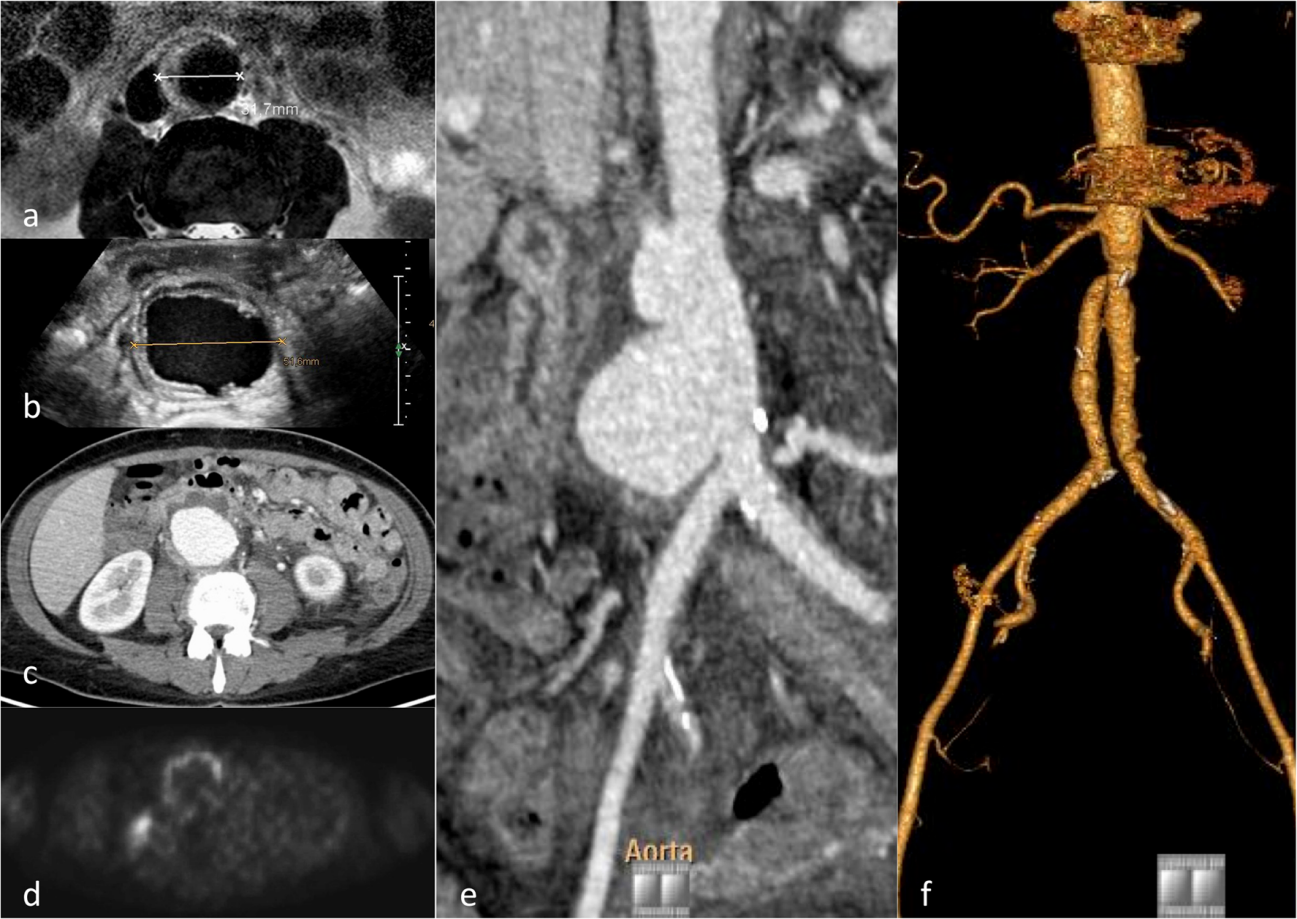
Mycotic abdominal aortic aneurysm. The 51-year-old female patient presented with abdominal/lumbar discomfort and sub-febrile temperatures. A lumbar MRI demonstrated no spondylodiscitis and a small abdominal aortic aneurysm with 35 mm diameter. One month later the abdominal aortic diameter had grown to 52 mm (b–c) and PET-CT (c–e) demonstrated wall thickening and PET uptake in the aortic wall and an atypical eccentric aneurysm presentation in coronal reformations (e). This was the only spot of infectious arthritis. Endocarditis of the mitral valve was demonstrated to be the focus. Additionally infectious arthritis of the left AC joint was treated by resection. Surgical reconstruction with deep vein (f) was performed; surgery confirmed a contained rupture and an aorto-duodenal fistulae
